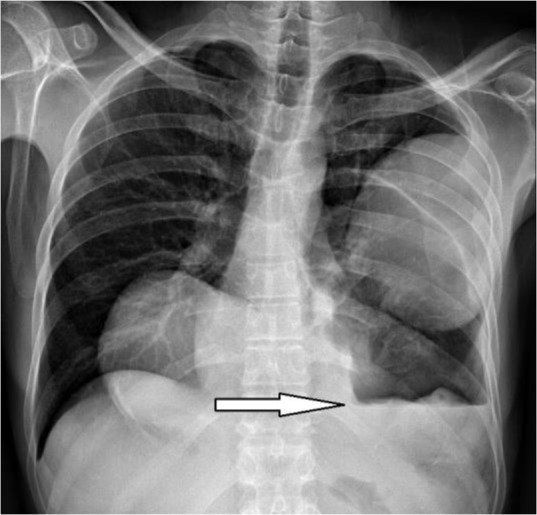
Chest X-ray shows left lung ruptured cyst (arrow).
